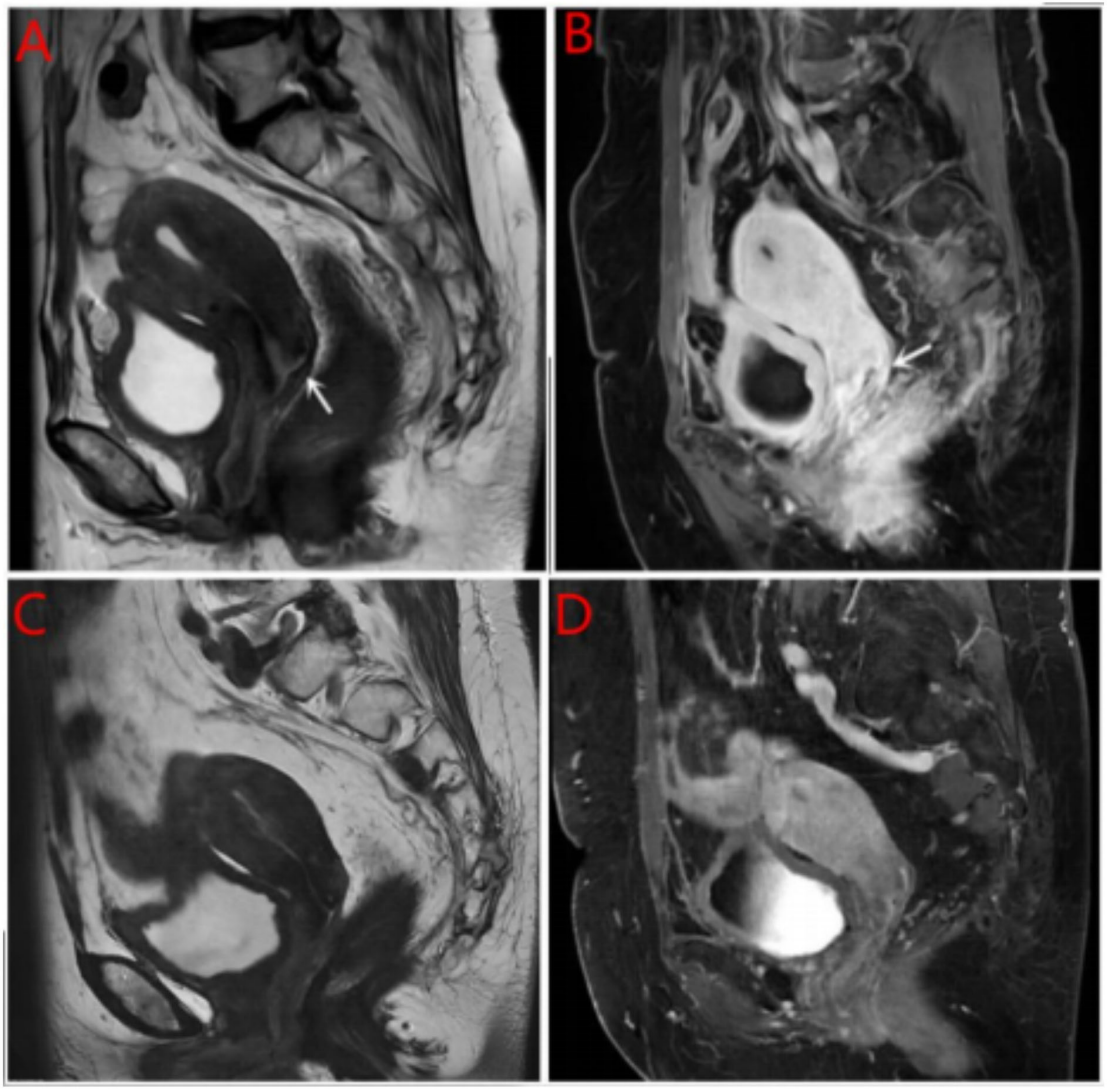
MRI cervical mass. (A) Pretreatment with MRI T2W1 imaging of the cervical mass; (B) Pretreatment with enhanced MRI imaging of the cervical mass; (C) Post treatment with MRI T2W1 imaging of the cervical mass; (D) Post treatment with enhanced MRI imaging of the cervical mass.
